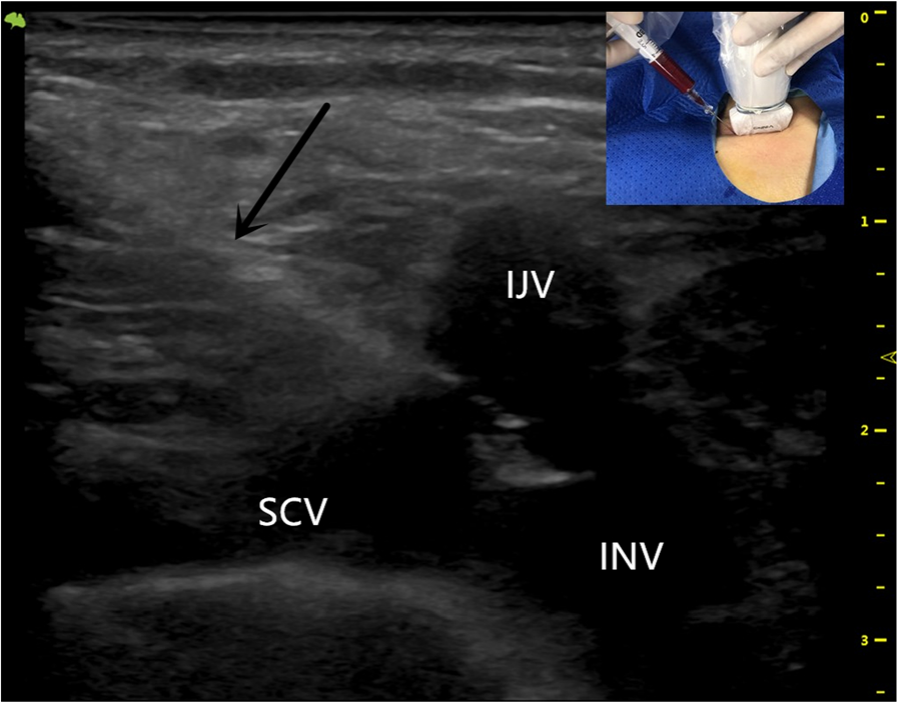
Ultrasound-guided successful puncture of right INV with inserting needle (black arrow). INV longitudinal view, in-plane approach. INV indicates innominate vein; IJV indicates internal jugular vein; SCV indicates the subclavian vein
